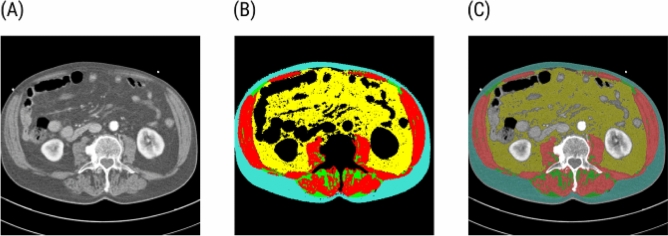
Segmentation of the CT L3 scan cross-section. (A) An example of a raw CT cross-section at the third lumbar vertebra level. (B) The segmentation map consists of the skeletal muscle tissue (red), intramuscular adipose tissue (green), visceral adipose tissue (yellow), and subcutaneous adipose tissue (turquoise). The black areas are excluded from the analysis. (C) An overlay of the segmentation map with the CT cross-section.
